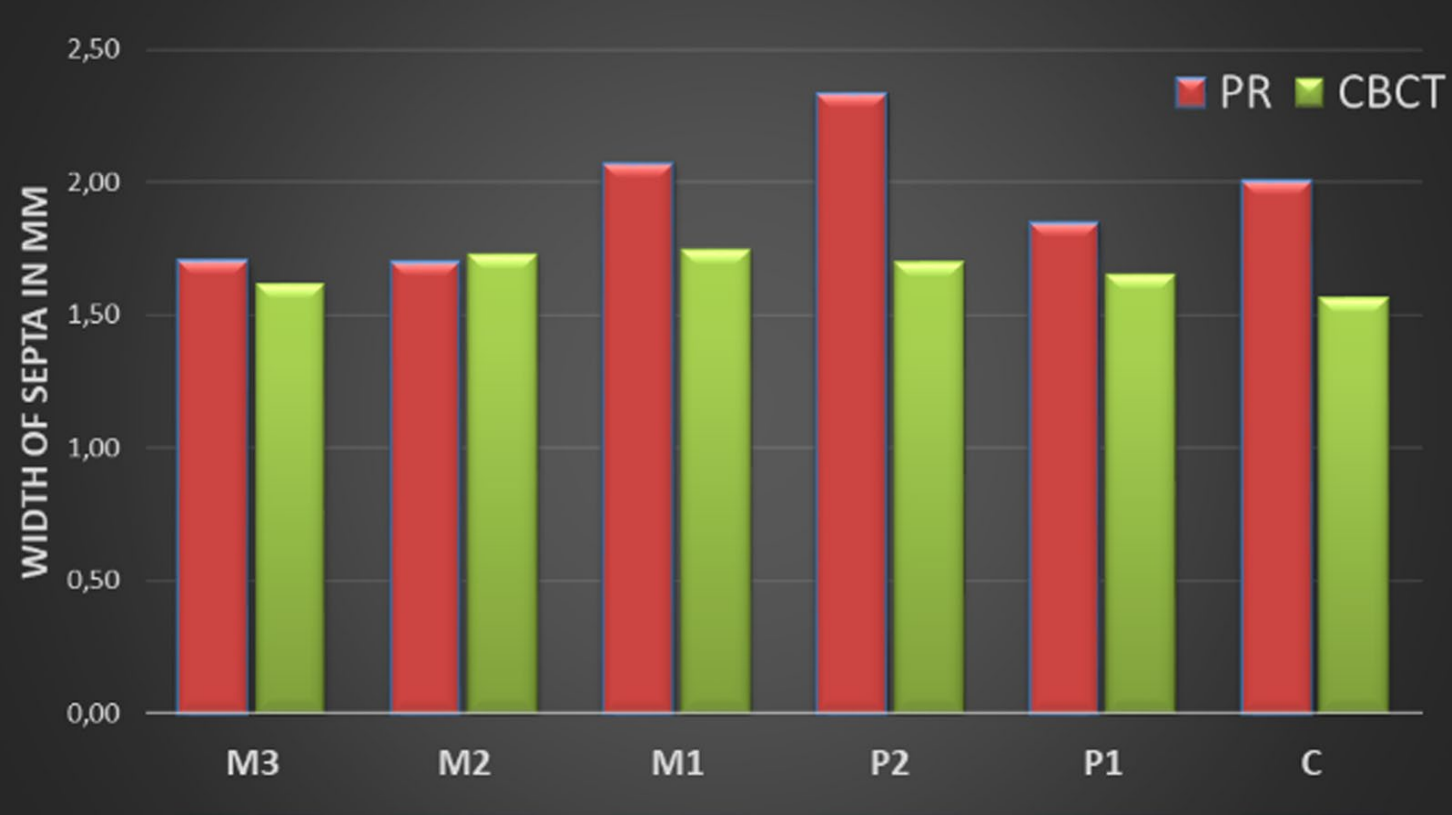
The width of the septa on PR versus CBCT images
